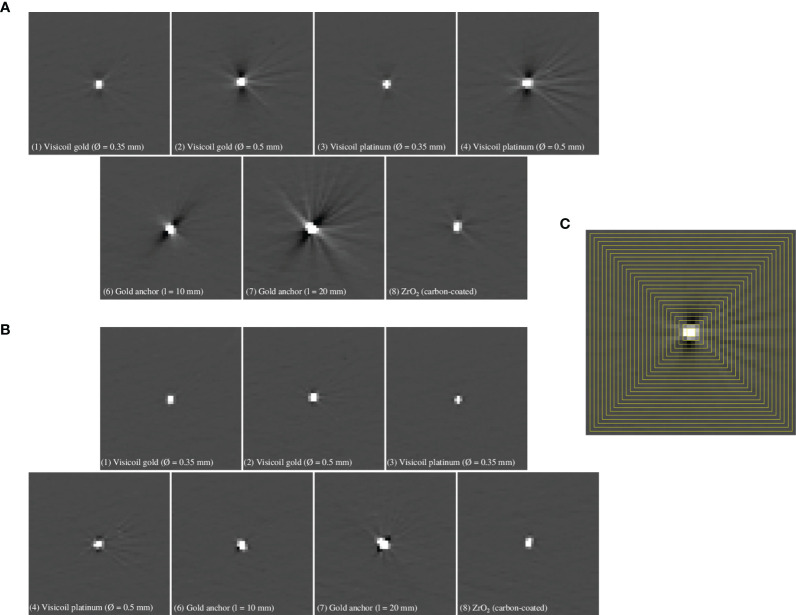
Reconstructed images for conventional treatment planning CT (A) and with iMAR correction (B) of 0.5mm slice thickness for 7 different fiducial markers (Table 1). (C) Square-shaped rings (yellow lines) used to determine the minimum and maximum values as a function of the distance from the marker, as explained in *Section 2.2.1*.
